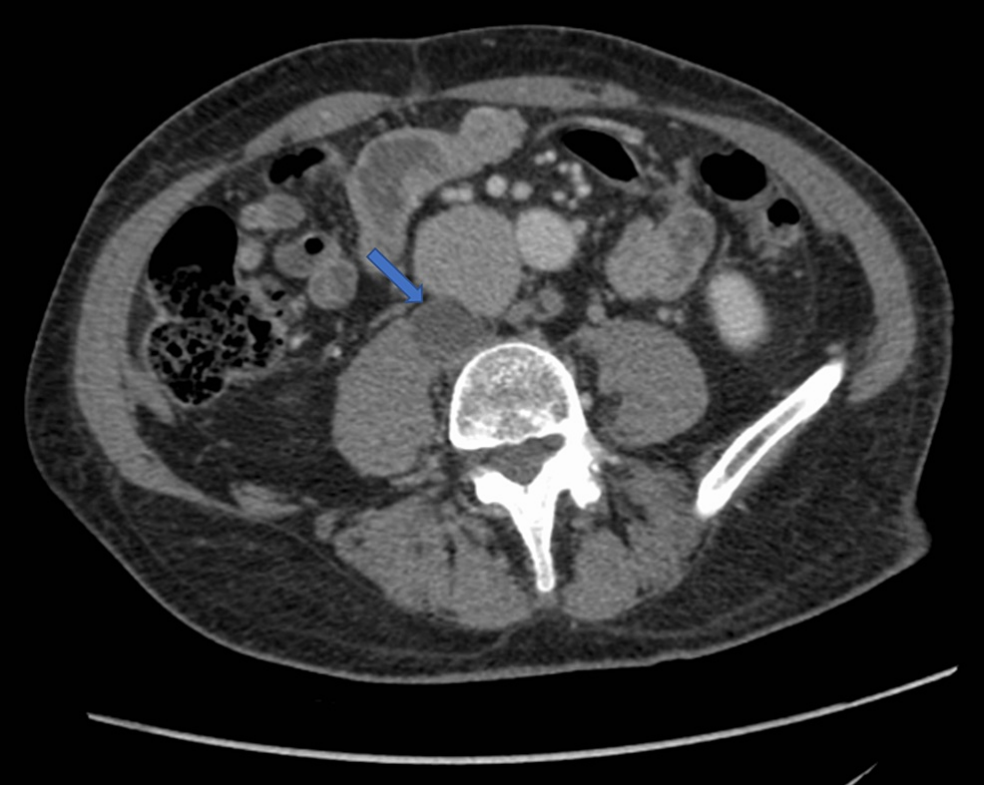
CT of the abdomen and pelvis with IV contrast The following CT scan image demonstrates an enlarged necrotic-appearing solitary nodule in the retrocaval region, posterior to the inferior vena cava and slightly to the right of the midline in the lower lumbar spine region (arrow). The nodule occupies the location of a lymph node and measures 28 × 24 mm. CT: computed tomography, IV: intravenous
